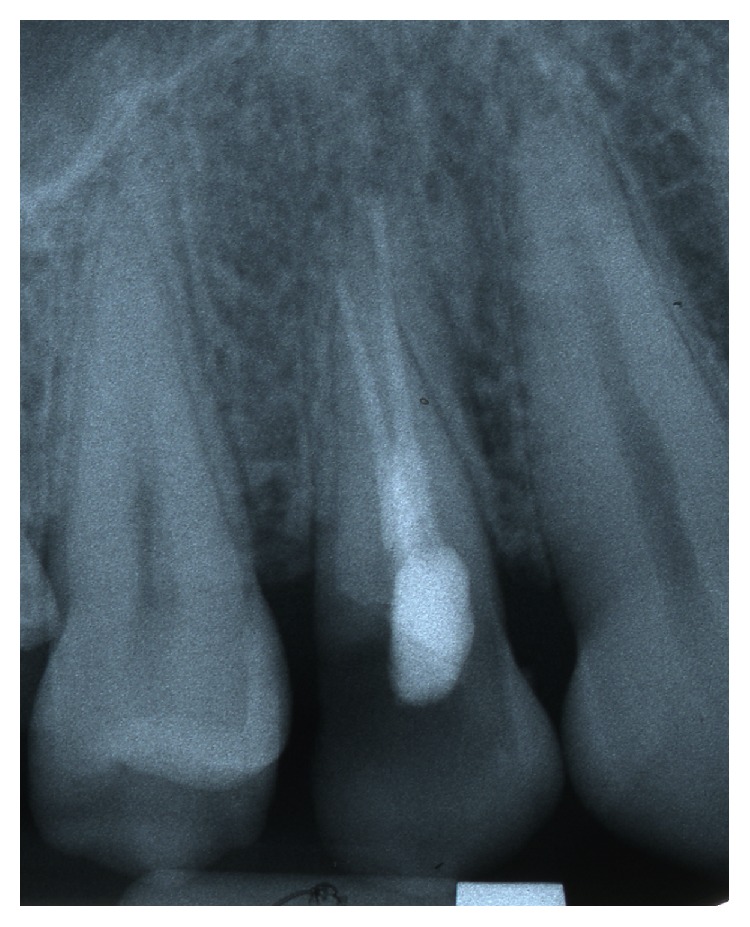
Periapical X-ray at baseline showing the tooth fracture and decay of the first right premolar.
